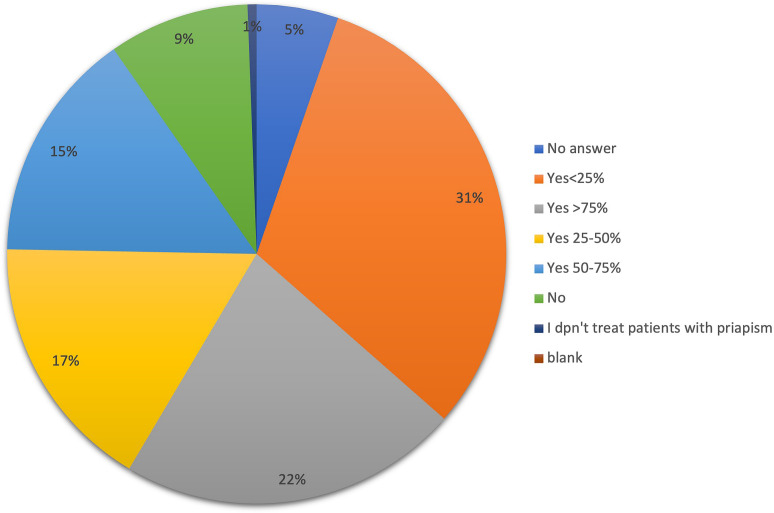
Penile color doppler ultrasound usage rate in the diagnosis of a patient with priapism.
